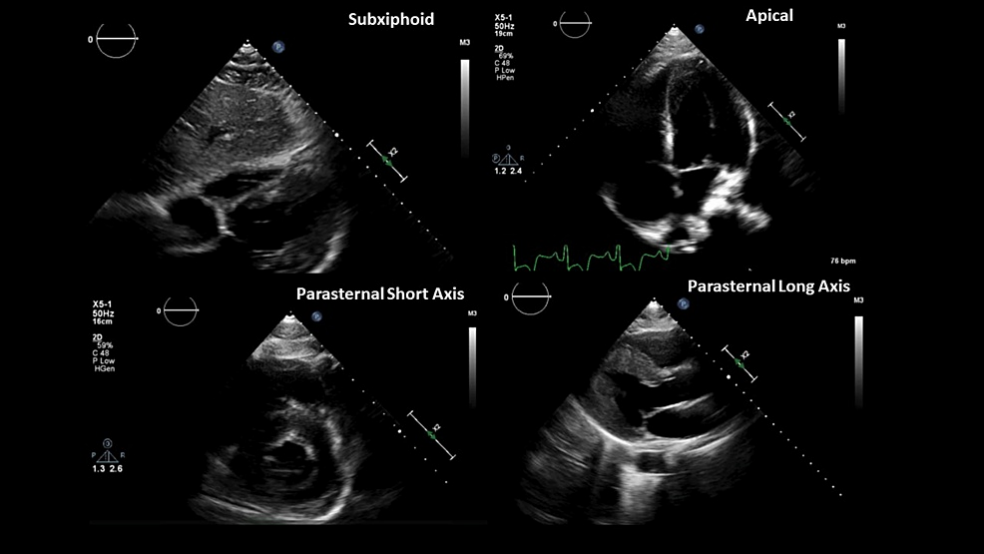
Two-dimensional echocardiogram showing severe concentric left ventricular hypertrophy.
